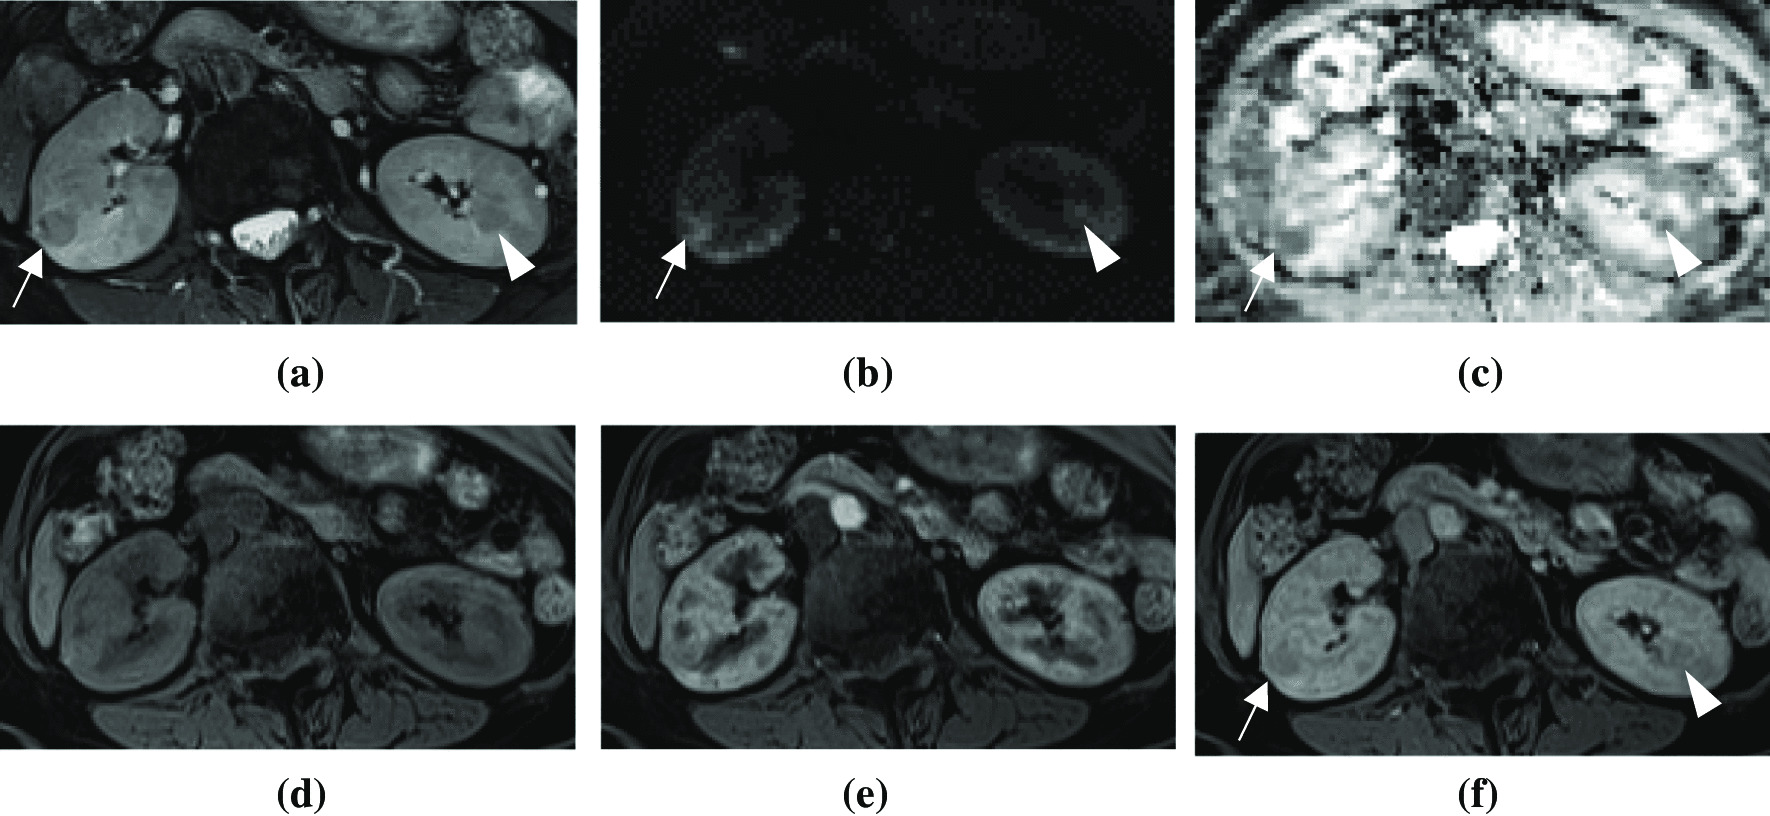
Abdominal MRI performed in August 2020. a On axial T2-weighted images, multiple papillary adenomas were observed in the renal cortices of the bilateral kidneys (arrow and arrowhead). Progression of the tumors was evident based on the increased tumor size and tumor number. b, c On axial diffusion-weighted images and apparent diffusion coefficient maps, more renal adenomas were noted in the bilateral kidneys with diffusion restriction (arrow and arrowhead). The imaging features remained consistent with those seen 10 years earlier. d, e, f On axial dynamic contrast-enhanced T1-weighted images with fat suppression, papillary adenomas present isointensity relative to the renal parenchyma on unenhanced images and relatively poor contrast enhancement in the arterial and venous phases (arrow and arrowhead). These imaging characteristics remained consistent with those seen 10 years earlier
